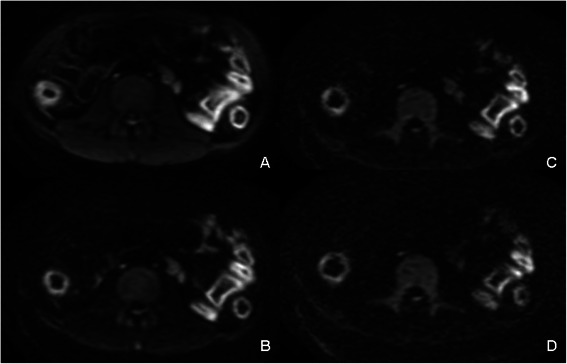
Qualitative image quality comparison among four b values. DWI images were obtained from a 30-year-old man. The Inflammatory lesion of approximal ileum was detected by endoscopy. When b = 800 s/mm^2^ was adopted, the lesion was demonstrated clearly as well as normal small intestinal wall, enlarged lymph nodes and abdominal wall structure (A). When b = 1500 s/mm^2^ was adopted, normal small intestinal wall could barely be identified (B) and when b = 2000 s/mm^2^ or b = 2500 s/mm^2^ was adopted (C and D), even abdominal wall structure became vague. The enlarged lymph nodes could not be identified when b = 2500 s/mm^2^ was adopted (D).
